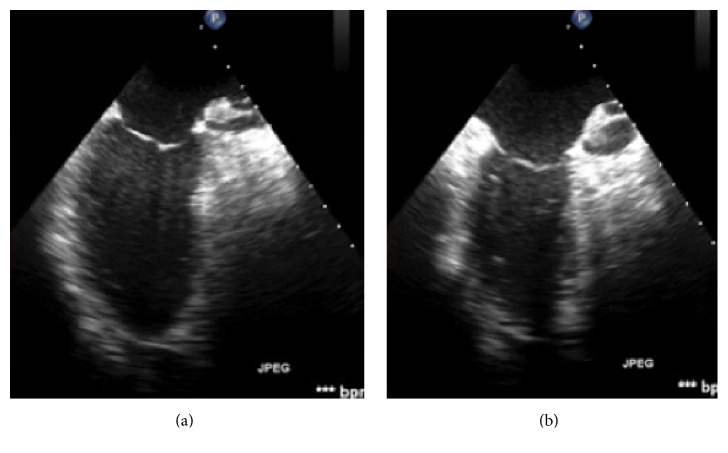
Transesophageal echocardiogram while on pentobarbital infusion, left ventricle ejection fraction of 20%: (a) end diastole; (b) end systole.
